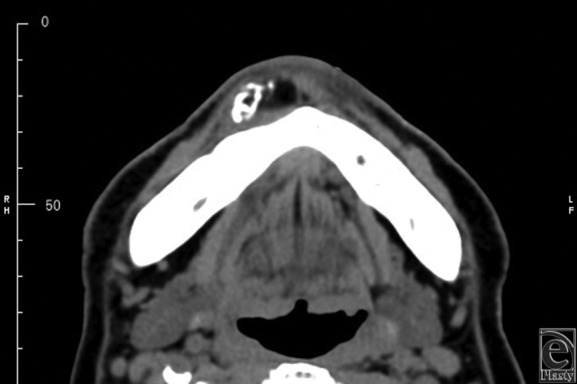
Computed tomographic scan shows a smooth mass of partial calcification at the mental region.
